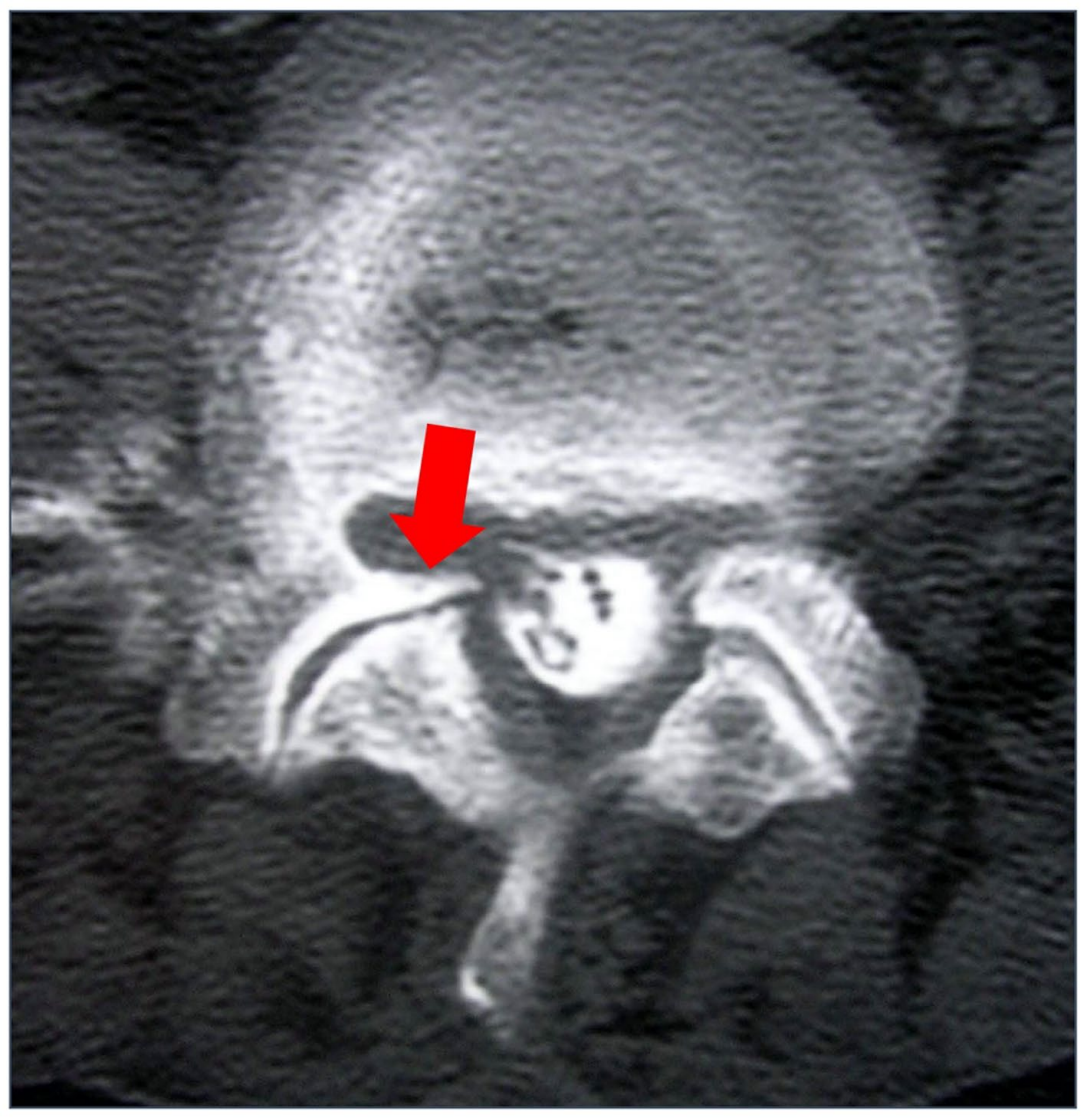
Myelography computed tomography (CT) in lumbar axial view. The red arrow indicates facet degeneration and osteophyte. This is a case from our institution.
